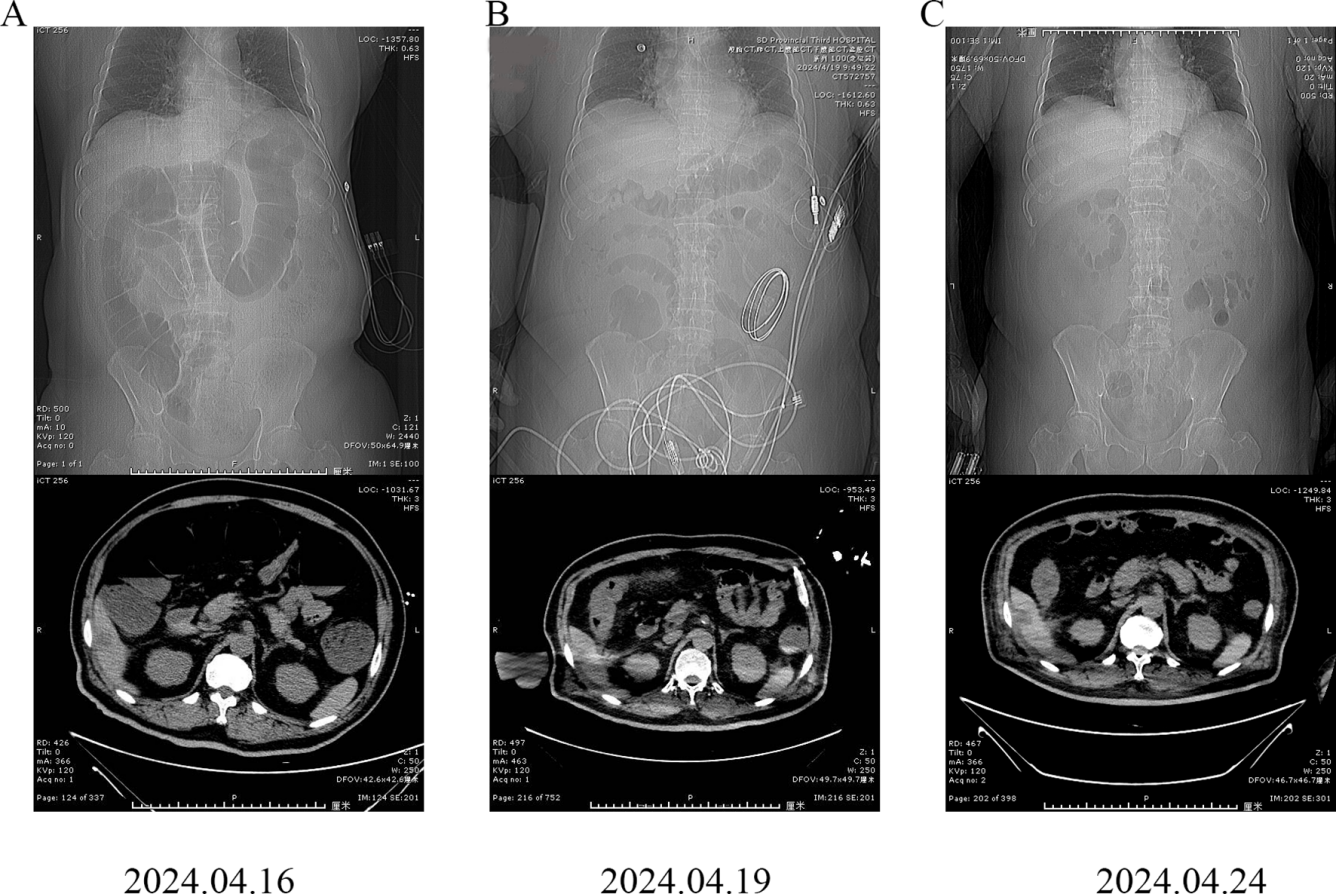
Abdominal computed tomography (CT) images of the patient. (A) April 16, 2024; (B) April 19, 2024; (C) April 24, 2024.
